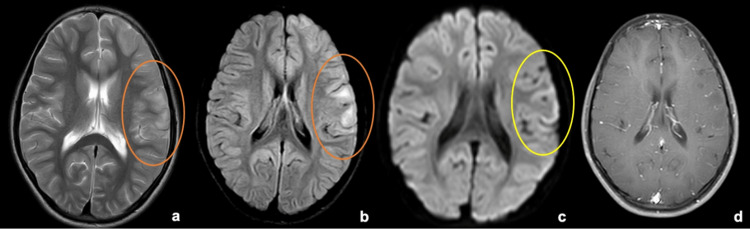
MRI brain at the upper section of the internal capsule From left to right: (a) T2W, (b) FLAIR, (c) DWI, (d) Post-contrast T1W images. Areas within the orange ellipse in (a) and (b) show focal T2/FLAIR hyperintensities in the left frontal cortical and juxta/subcortical regions with gyral swelling and sulcal effacement. The yellow ellipse marked in the DWI image (c) shows corresponding areas of cortical restriction. There is no significant post-contrast enhancement in this section (d). DWI - Diffusion-weighted imaging; FLAIR - Fluid attenuated inversion recovery; MRI - Magnetic resonance imaging; T1W - T1 weighted; T2W - T2 weighted
